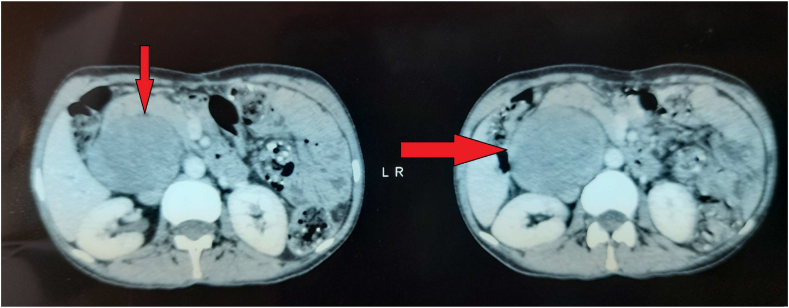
Abdomino-pelvic computed tomography demonstrates a retroperitoneal mass developed in anteraortocaval region measuring 55 × 65 x 88 (arrow).
